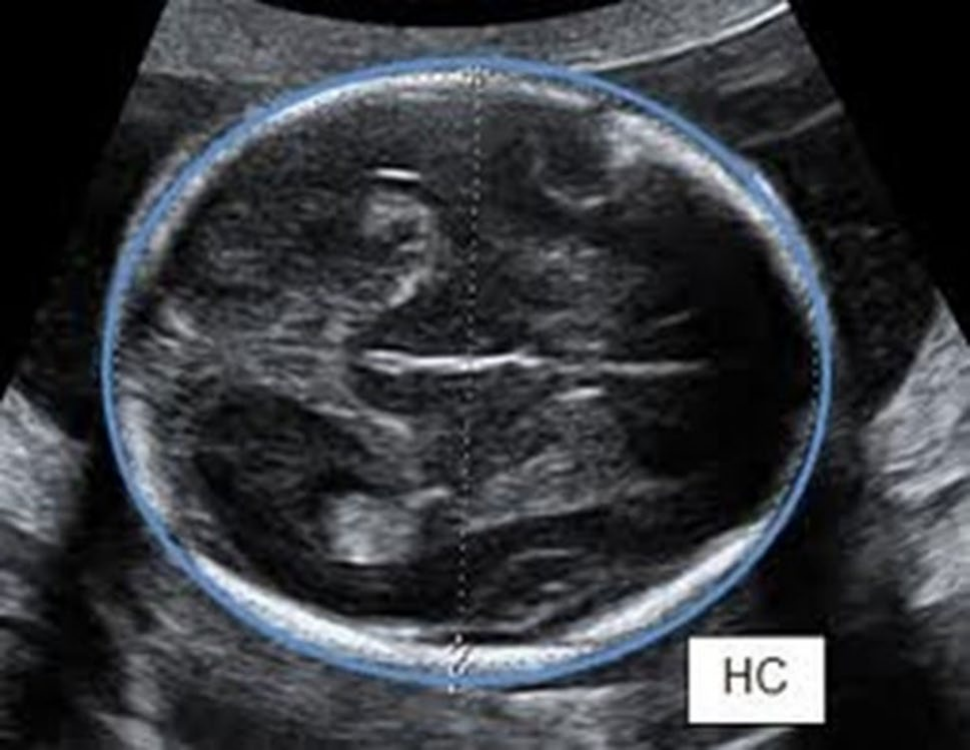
Ultrasonographic image of measuring fetal head circumference
